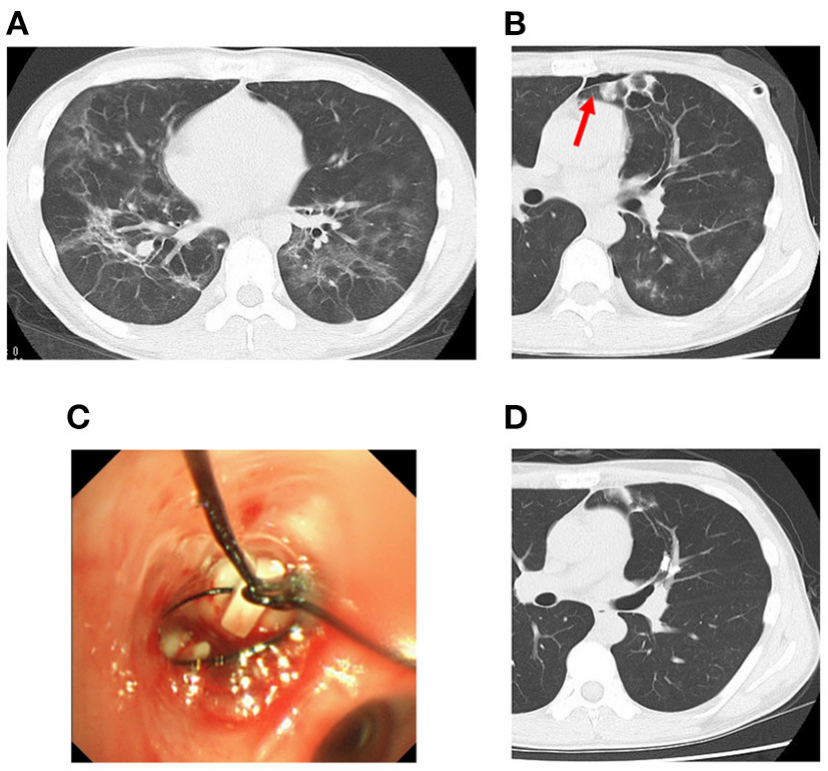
Chest computed tomography (CT) scan on admission (A), at 11 days (B), and after bronchial occlusion with a endobronchial Watanabe spigot (EWS) (D), and EWS (C). CT scan of the chest showing diffuse ground glass opacity on admission (A). CT scan of the chest showing cysts clustered on the peripheral side of the left B4 at 11 days (B). EWS shown being implanted in left B4b (C). And, CT scan showing improvement in the pneumothorax after bronchial occlusion with EWS (D).
